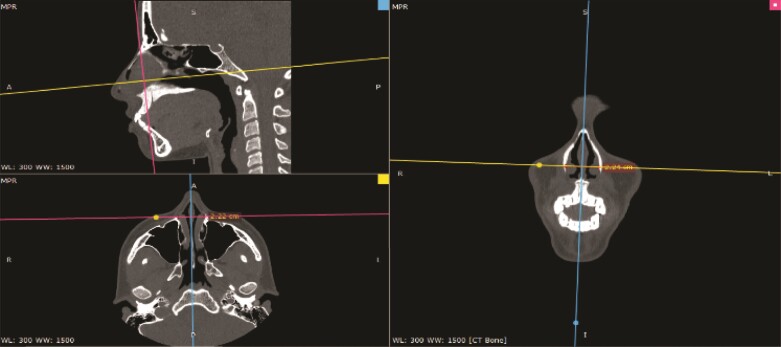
Postoperative evaluation. Postoperative computed tomography scan measure of the distance between inferior turbinate’s heads in Radiant’s software multiplanar reconstruction mode.
